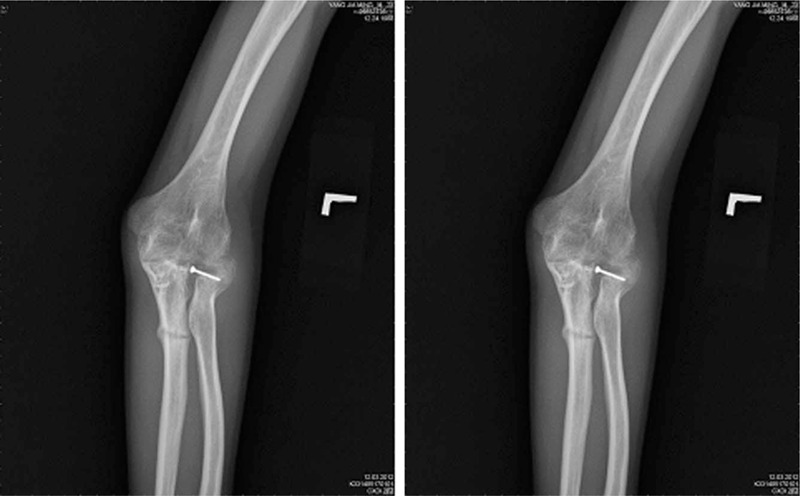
Callus and increased density of ulnar shaft was identified on the subsequent X-rays.
